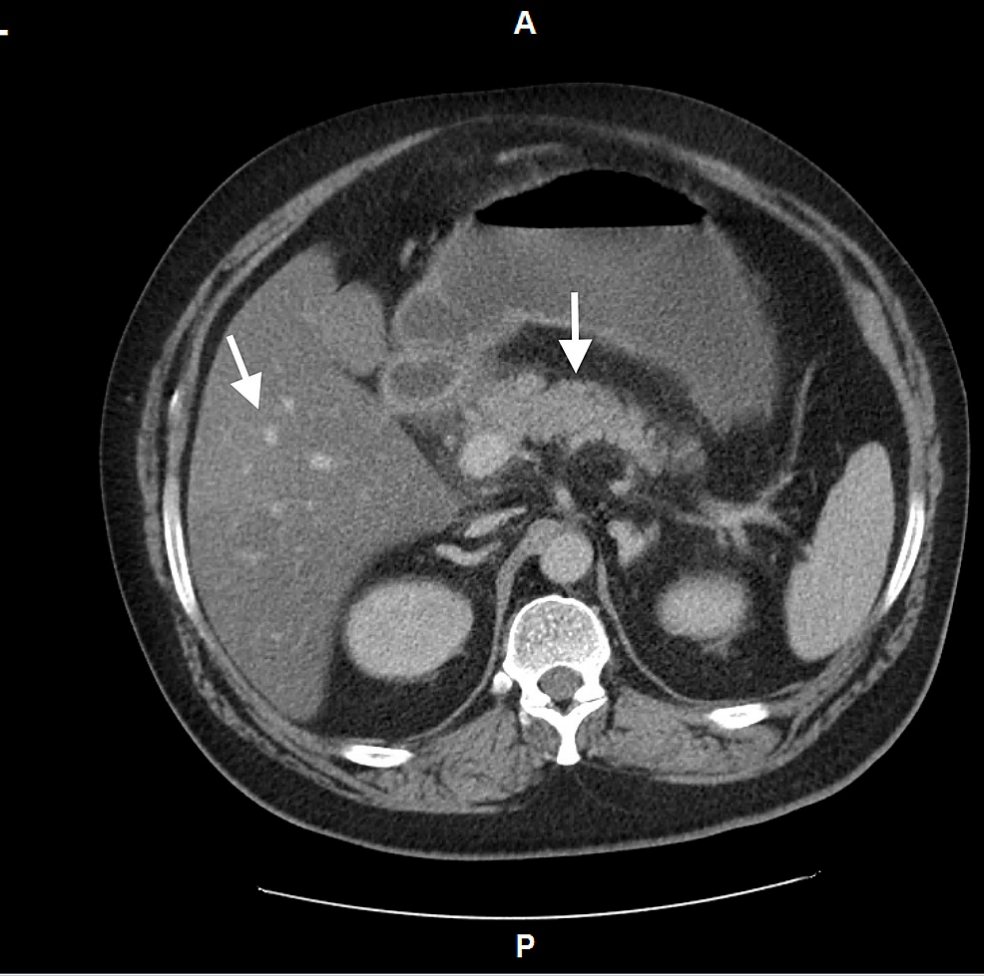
CT abdomen/pelvis with contrast exhibiting mild pancreatitis with hepatic steatosis.
